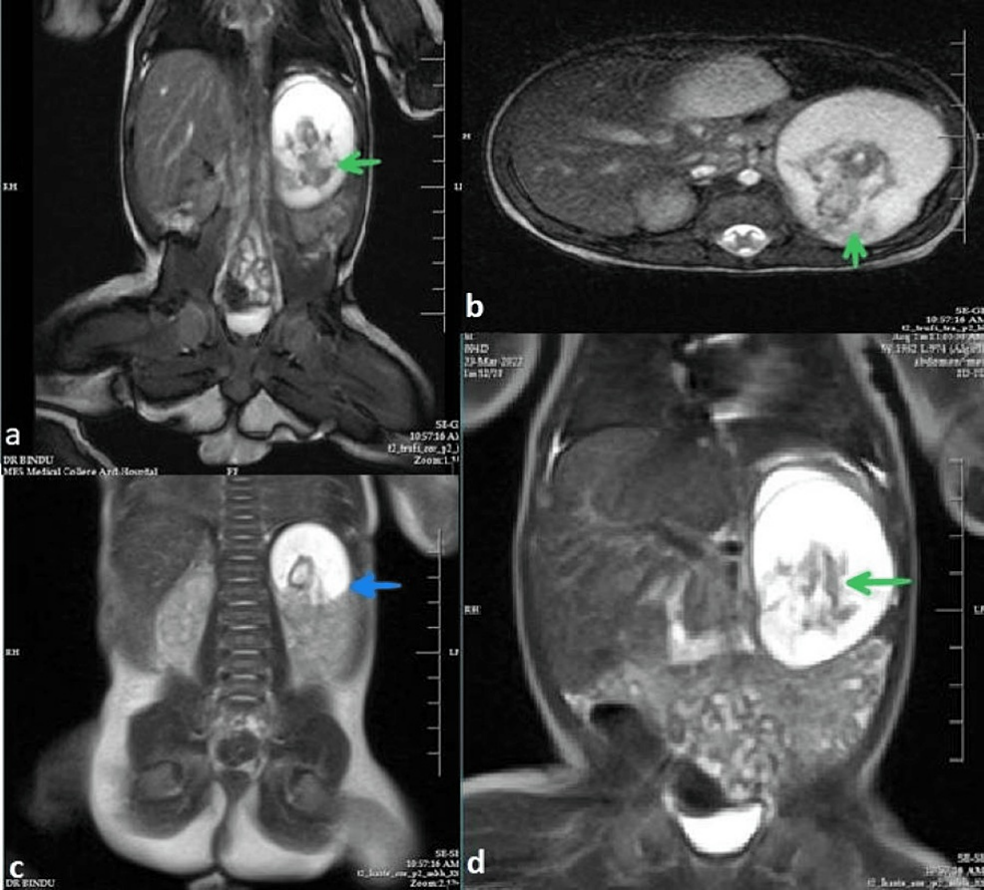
Coronal (a) and axial (b) TRUFI and coronal HASTE (d) 1.5 T MR images of the abdomen showed a well-defined, lobulated, intra-abdominal retroperitoneal mass in the left suprarenal region with mixed high and low intensities and predominant cystic consistency. T2 hypointense areas resembling fetal parts (limbs, head, abdomino-thoracic cavity) were seen within the lesion (green arrow). Coronal HASTE (c) image showed the mass abutting the left kidney (blue arrow) and spleen. TRUFI - True Fast Imaging with steady-state-free precession HASTE - Half-Fourier acquisition single-shot turbo SE
